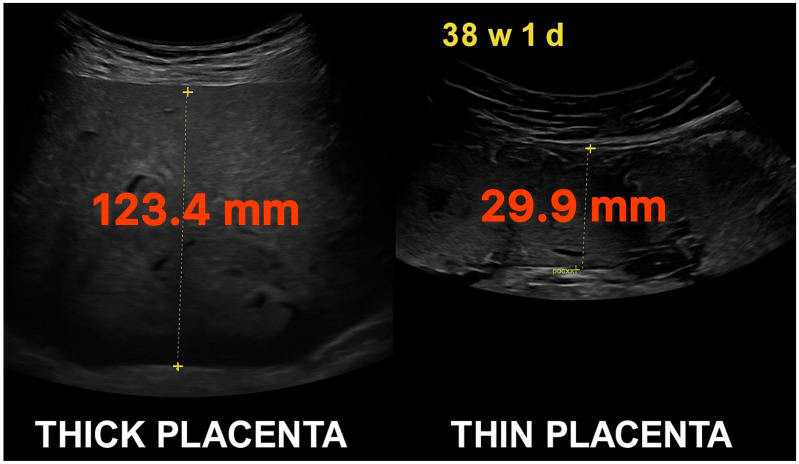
Comparative ultrasonographic images of placental thickness at 38 weeks and 1 day of gestation. Left: markedly thick placenta measuring 123.4 mm. Right: thin placenta measuring 29.9 mm. Both measurements were taken from the anterior uterine wall using standardized perpendicular caliper placement.
